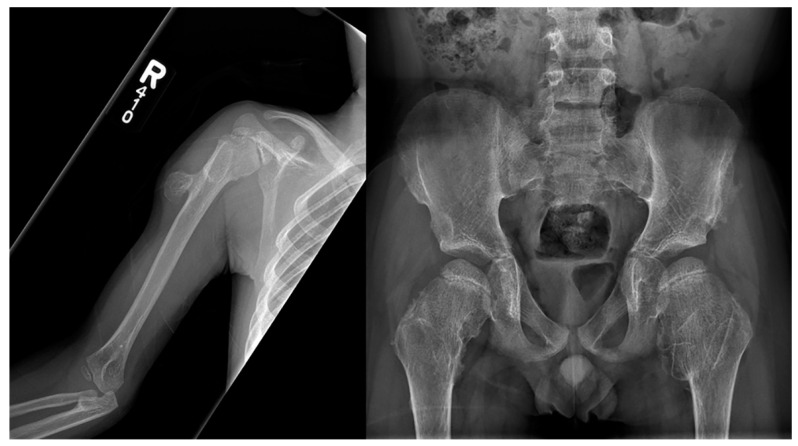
Frontal radiography of the right humerus and pelvis in a patient with confirmed Langer-Giedion syndrome show multiple exostoses along the proximal right humeral shaft, both femoral necks, and both iliac bones. The bones also appear demineralized.
